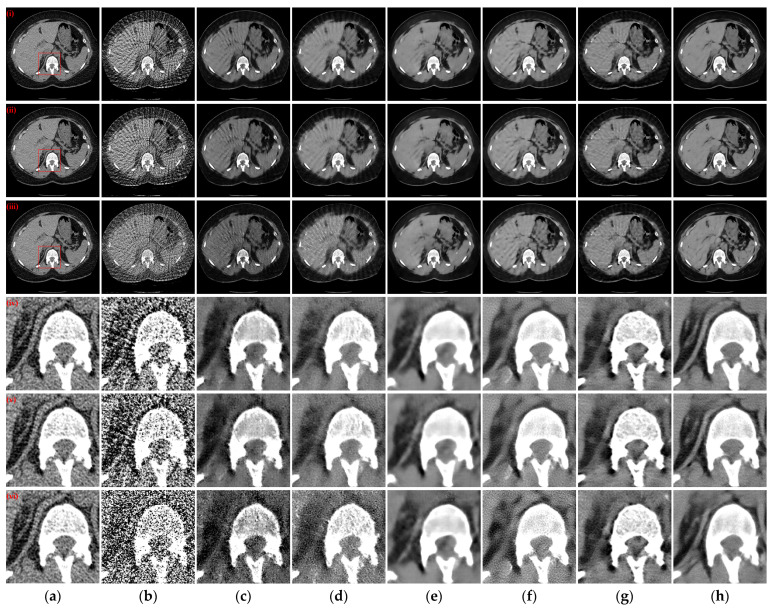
The SVCT reconstruction results from different methods for different intensity levels of mixed noise with 64 projection views. (a) The reference image; (b) FBP; (c) MWNet; (d) DuDoTrans; (e) DDPTransformer; (f) MIST; (g) RegFormer; (h) Ours. (i)–(iii) are the reconstructed CT images with Poisson noise levels of 1 × 10^6^, 5 × 10^5^, and 1 × 10^5^, respectively, and (iv)–(vi) correspond to the enlarged ROIs marked by the red boxes in the reference images above. The display window is [−160, 240] HU.
